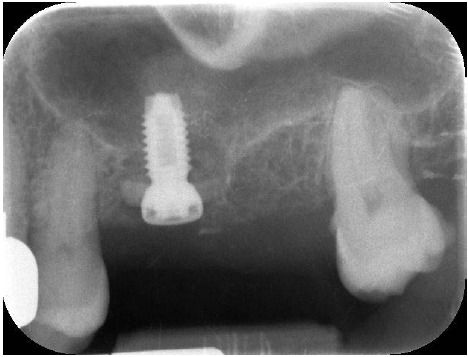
Periapical x-ray showing the implant and the graft in place after sutures.
